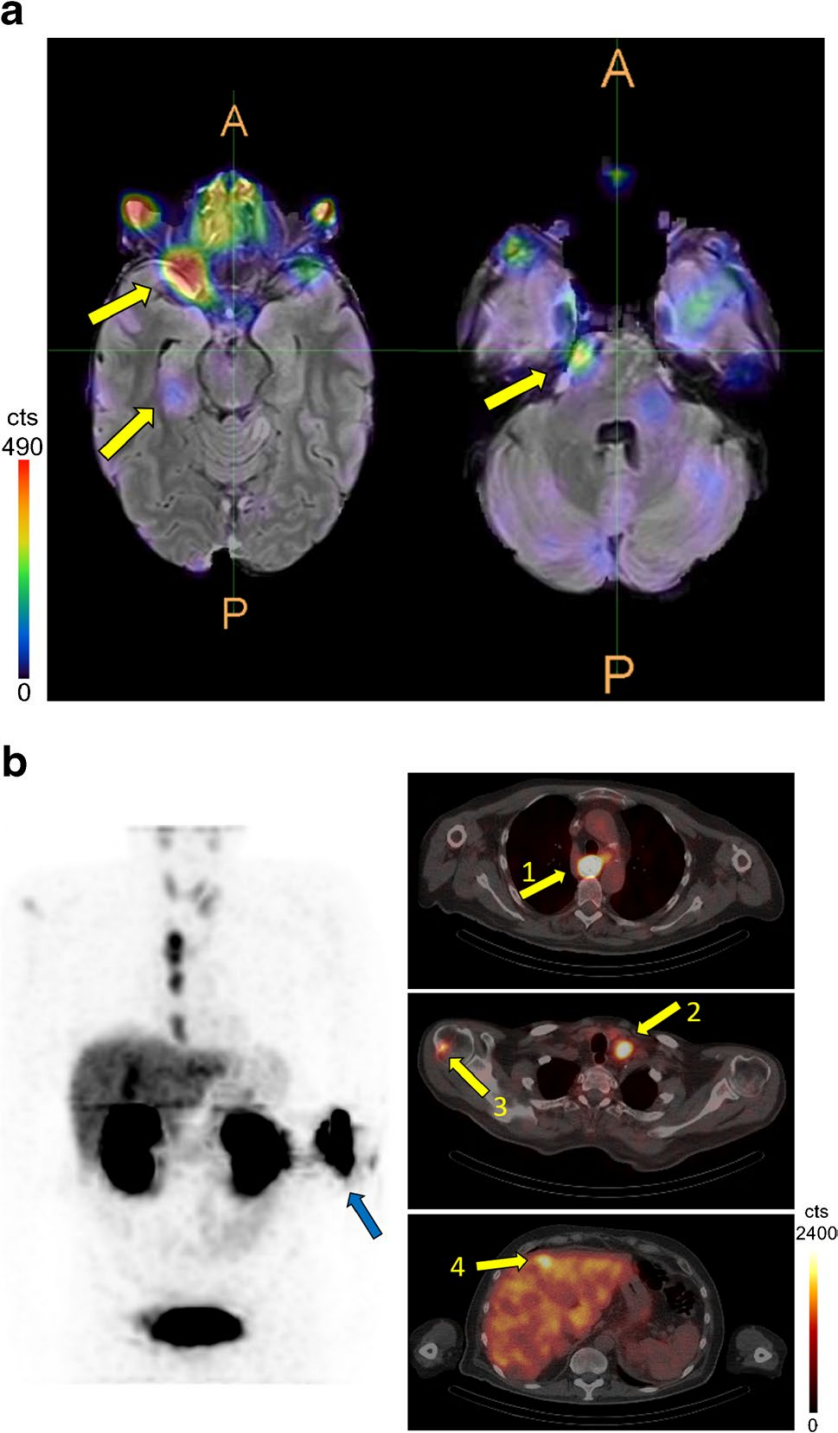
Uptake of RAD201 in a brain metastases (arrows) fused with individual MRI images from patient 2. b Patient 5 with esophageal cancer (1) and metastases in lymph node (2), bone (3), and liver (4). The blue arrow indicates the injection site
